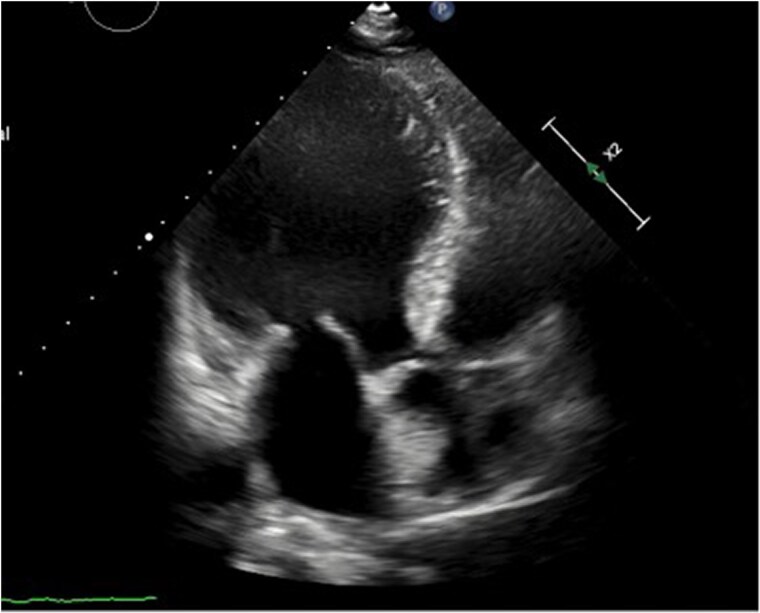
Pre-operative transthoracic echocardiogram. Three-chamber apical view showing a basal left ventricular aneurysm with thinned, dyskinetic wall segments.
